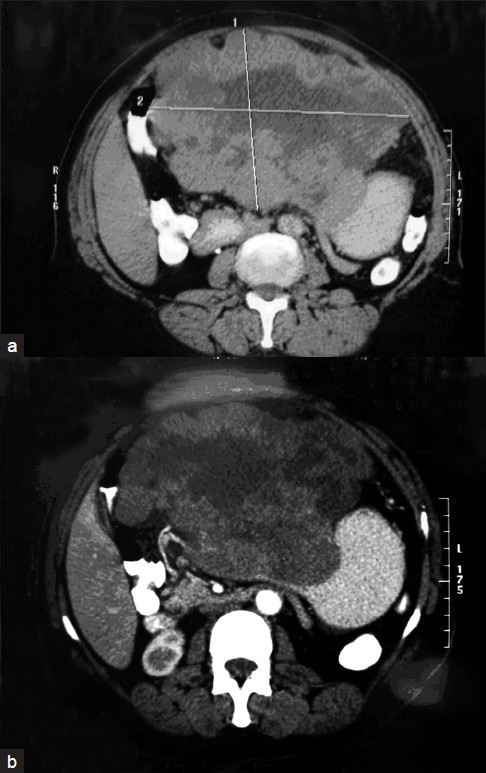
(a) Computed tomography (CT) for primary gastric gastrointestinal stromal tumors (GISTs); (b) CT for primary gastric GIST
